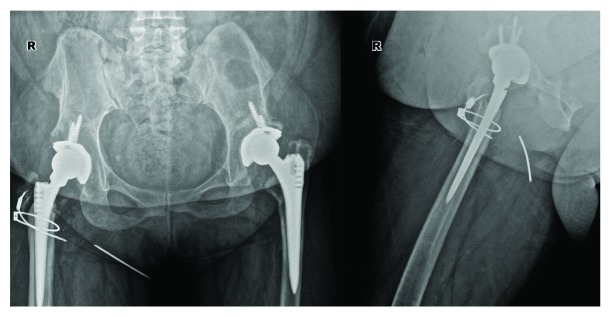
Postoperative 4-year pelvis anteroposterior and hip lateral radiographs of the patient showing medial migration of the broken cable part.
